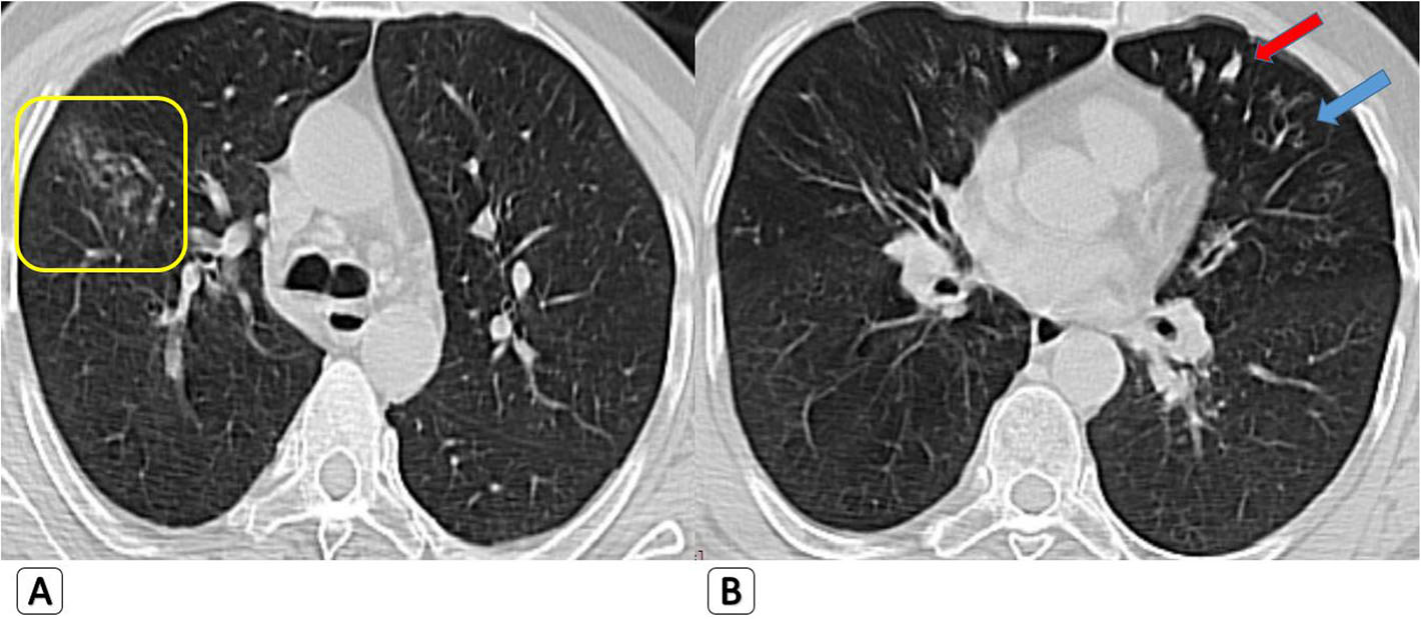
Mixed parenchymal/alveolar and airway abnormalities seen with H1N1: 37-year-old male H1N1 patient. a Axial HRCT chest lung window showing right sided mild alveolar ground glass nodular opacities (yellow square). b Axial HRCT lung window showing left lingular zone and to lesser extent right middle lobar air trapping with bronchiectatic changes (blue arrow) and mucous plugging (red arrow)
